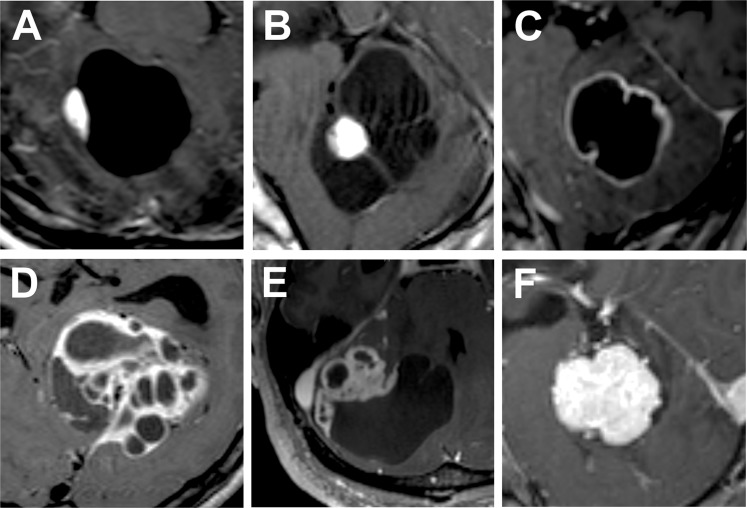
Gadolinium-enhanced T1W images of magnetic resonance imaging of hemangioblastomas (HBMs). Type Ce refers (A,B) HBMs of classical radiological features with a main cyst and a mural nodule. Purely cystic HBMs (C) used to be an independent subtype, however, they were classified as Type Ci together with HBMs with multiple intratumoral cysts (D). Tumors with mixed intratumoral and extratumoral cysts (E) were also identified (Type Cm). Solid tumor (F) was the second most common subtype of HBMs.
